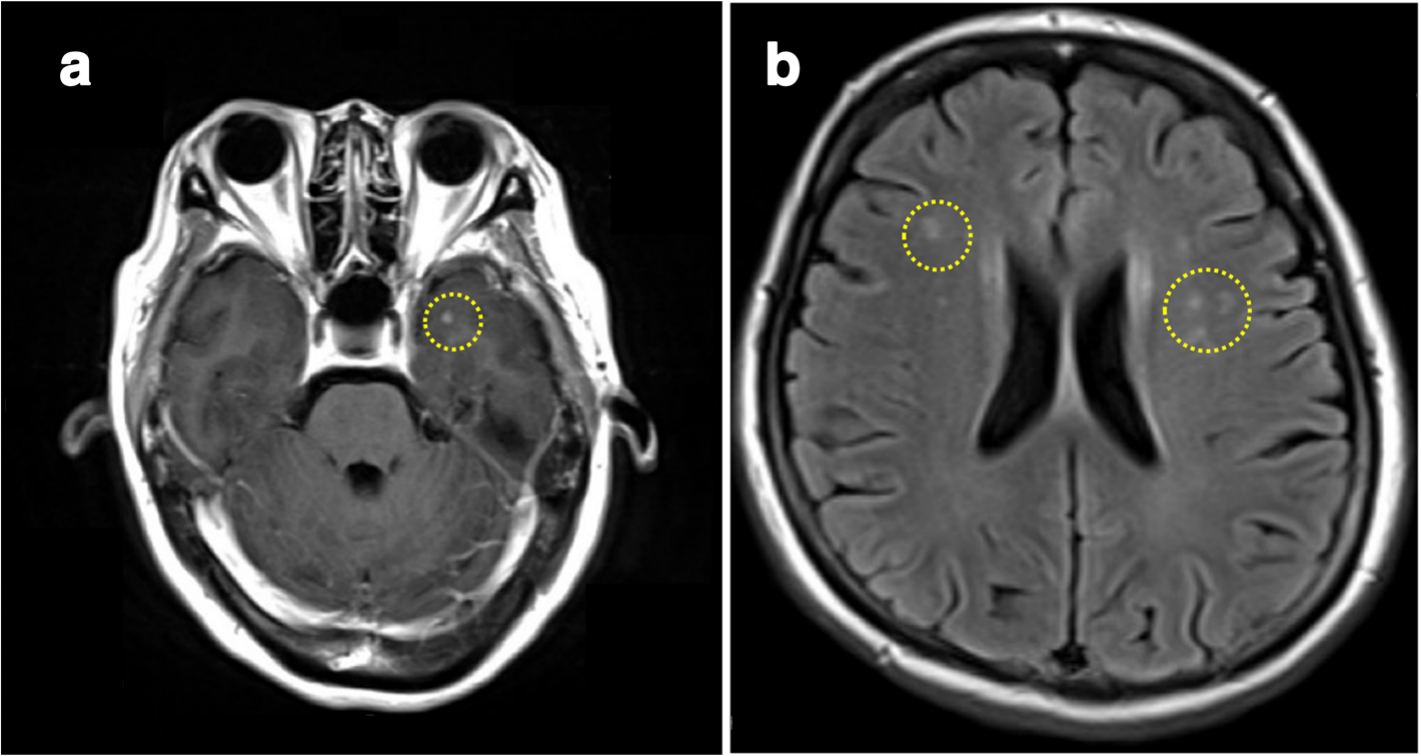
Gadolinium-enhanced magnetic resonance imaging scans of the brain. The images depict several small nodules in the left temporal lobe (a) and the subcortical white matter around both ventricles (b)
